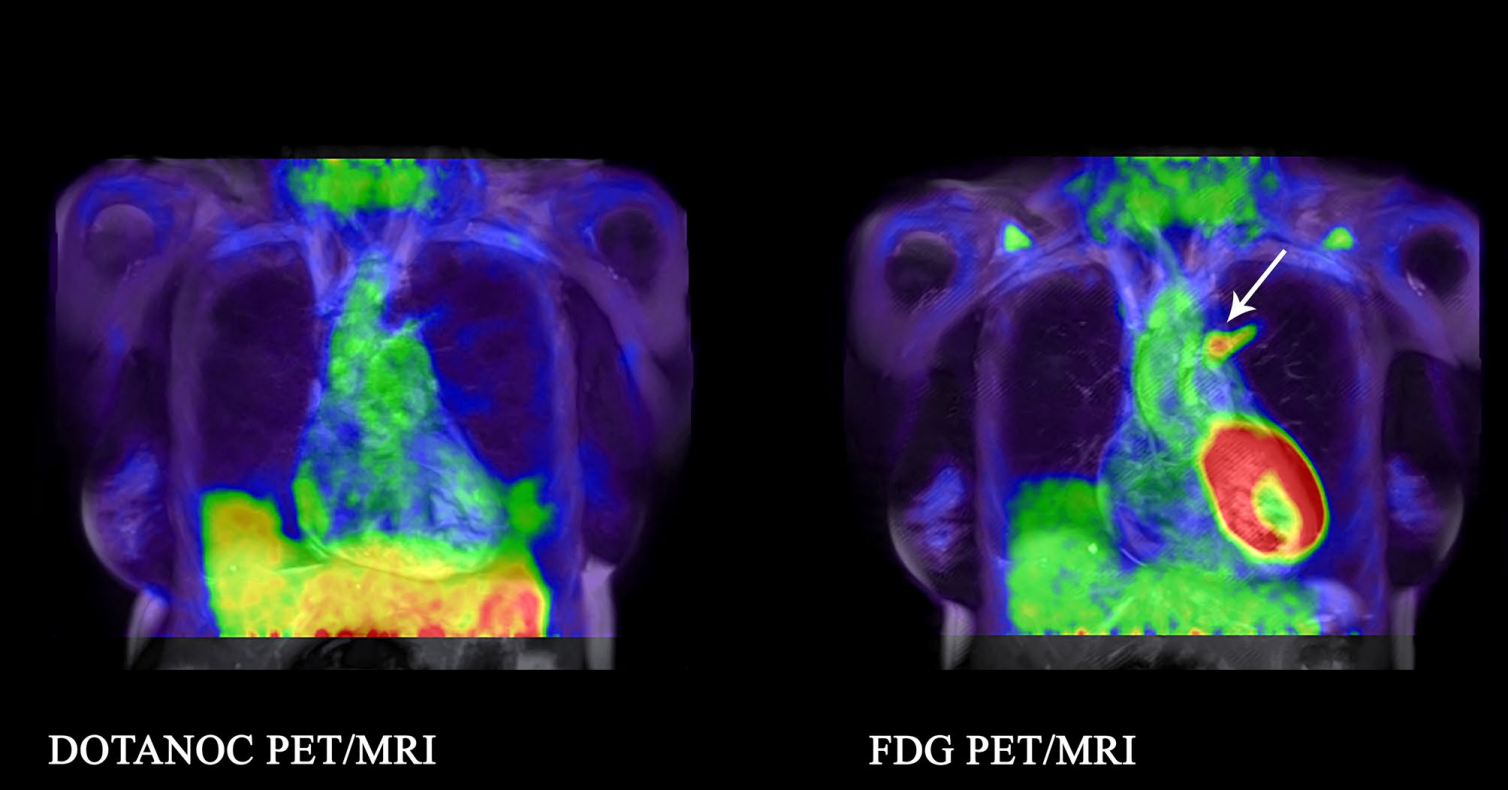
Lesion visualised in anterior segment of the left upper lobe adjacent to the aorta with FDG in 47-year-old female, IGRA-positive TB contact, taking isoniazid for 5 days. FDG SUVmax 5.50. No DOTANOC uptake.
